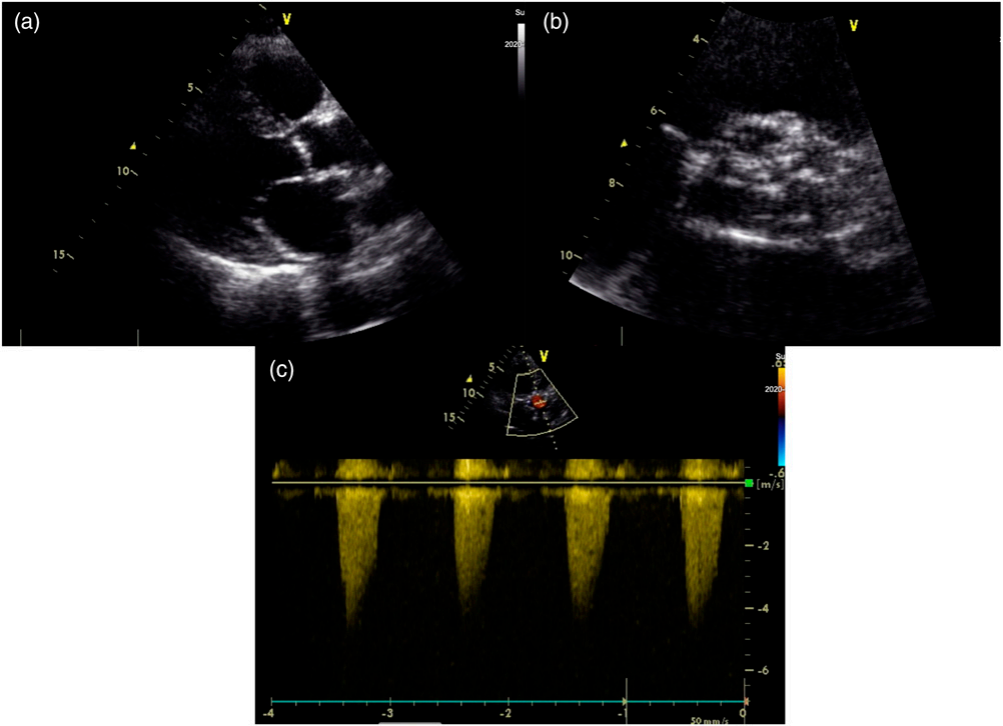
Preoperative echocardiography findings: (a) parasternal long-axis view showing reduced aortic valve leaflets motion, (b) parasternal short-axis view showing significant calcification of aortic valve leaflets, (c) peak aortic jet velocity measured by CW doppler.
